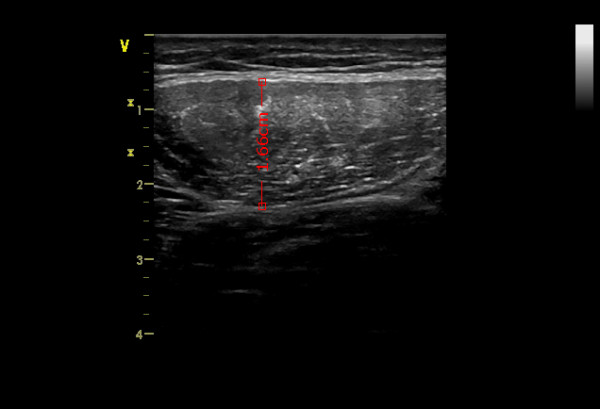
Image of the rectus femoris with indication of the diameter, obtained with ultrasound imaging.
